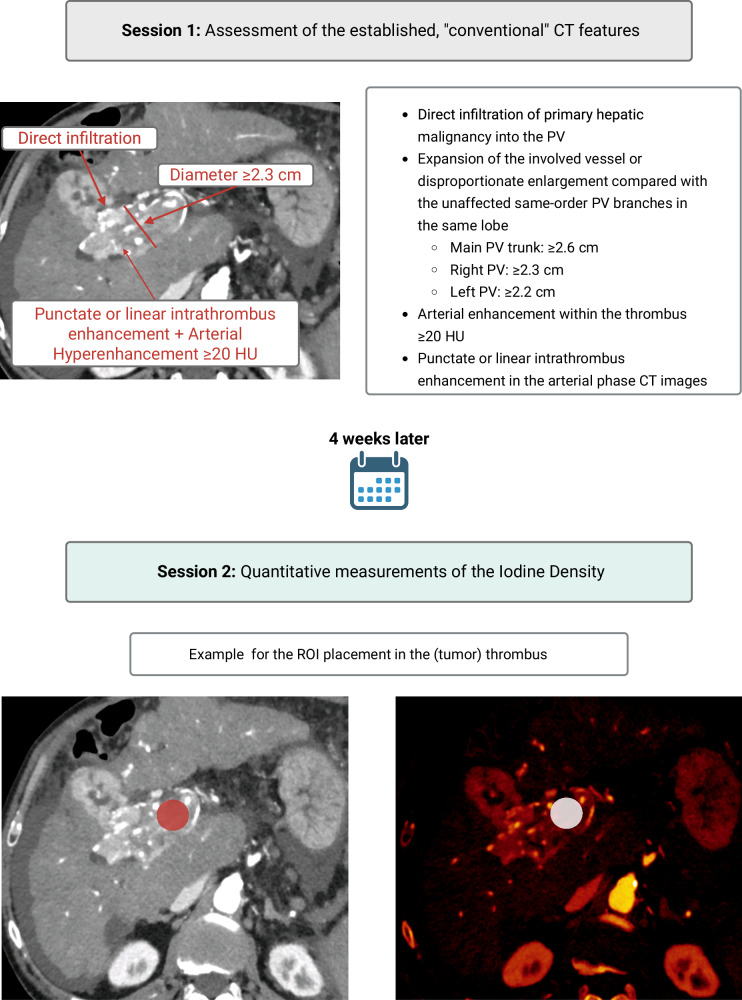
Overview of the established CT features [14] and an example of the ROI measurements. Created with BioRender. PV, portal vein
